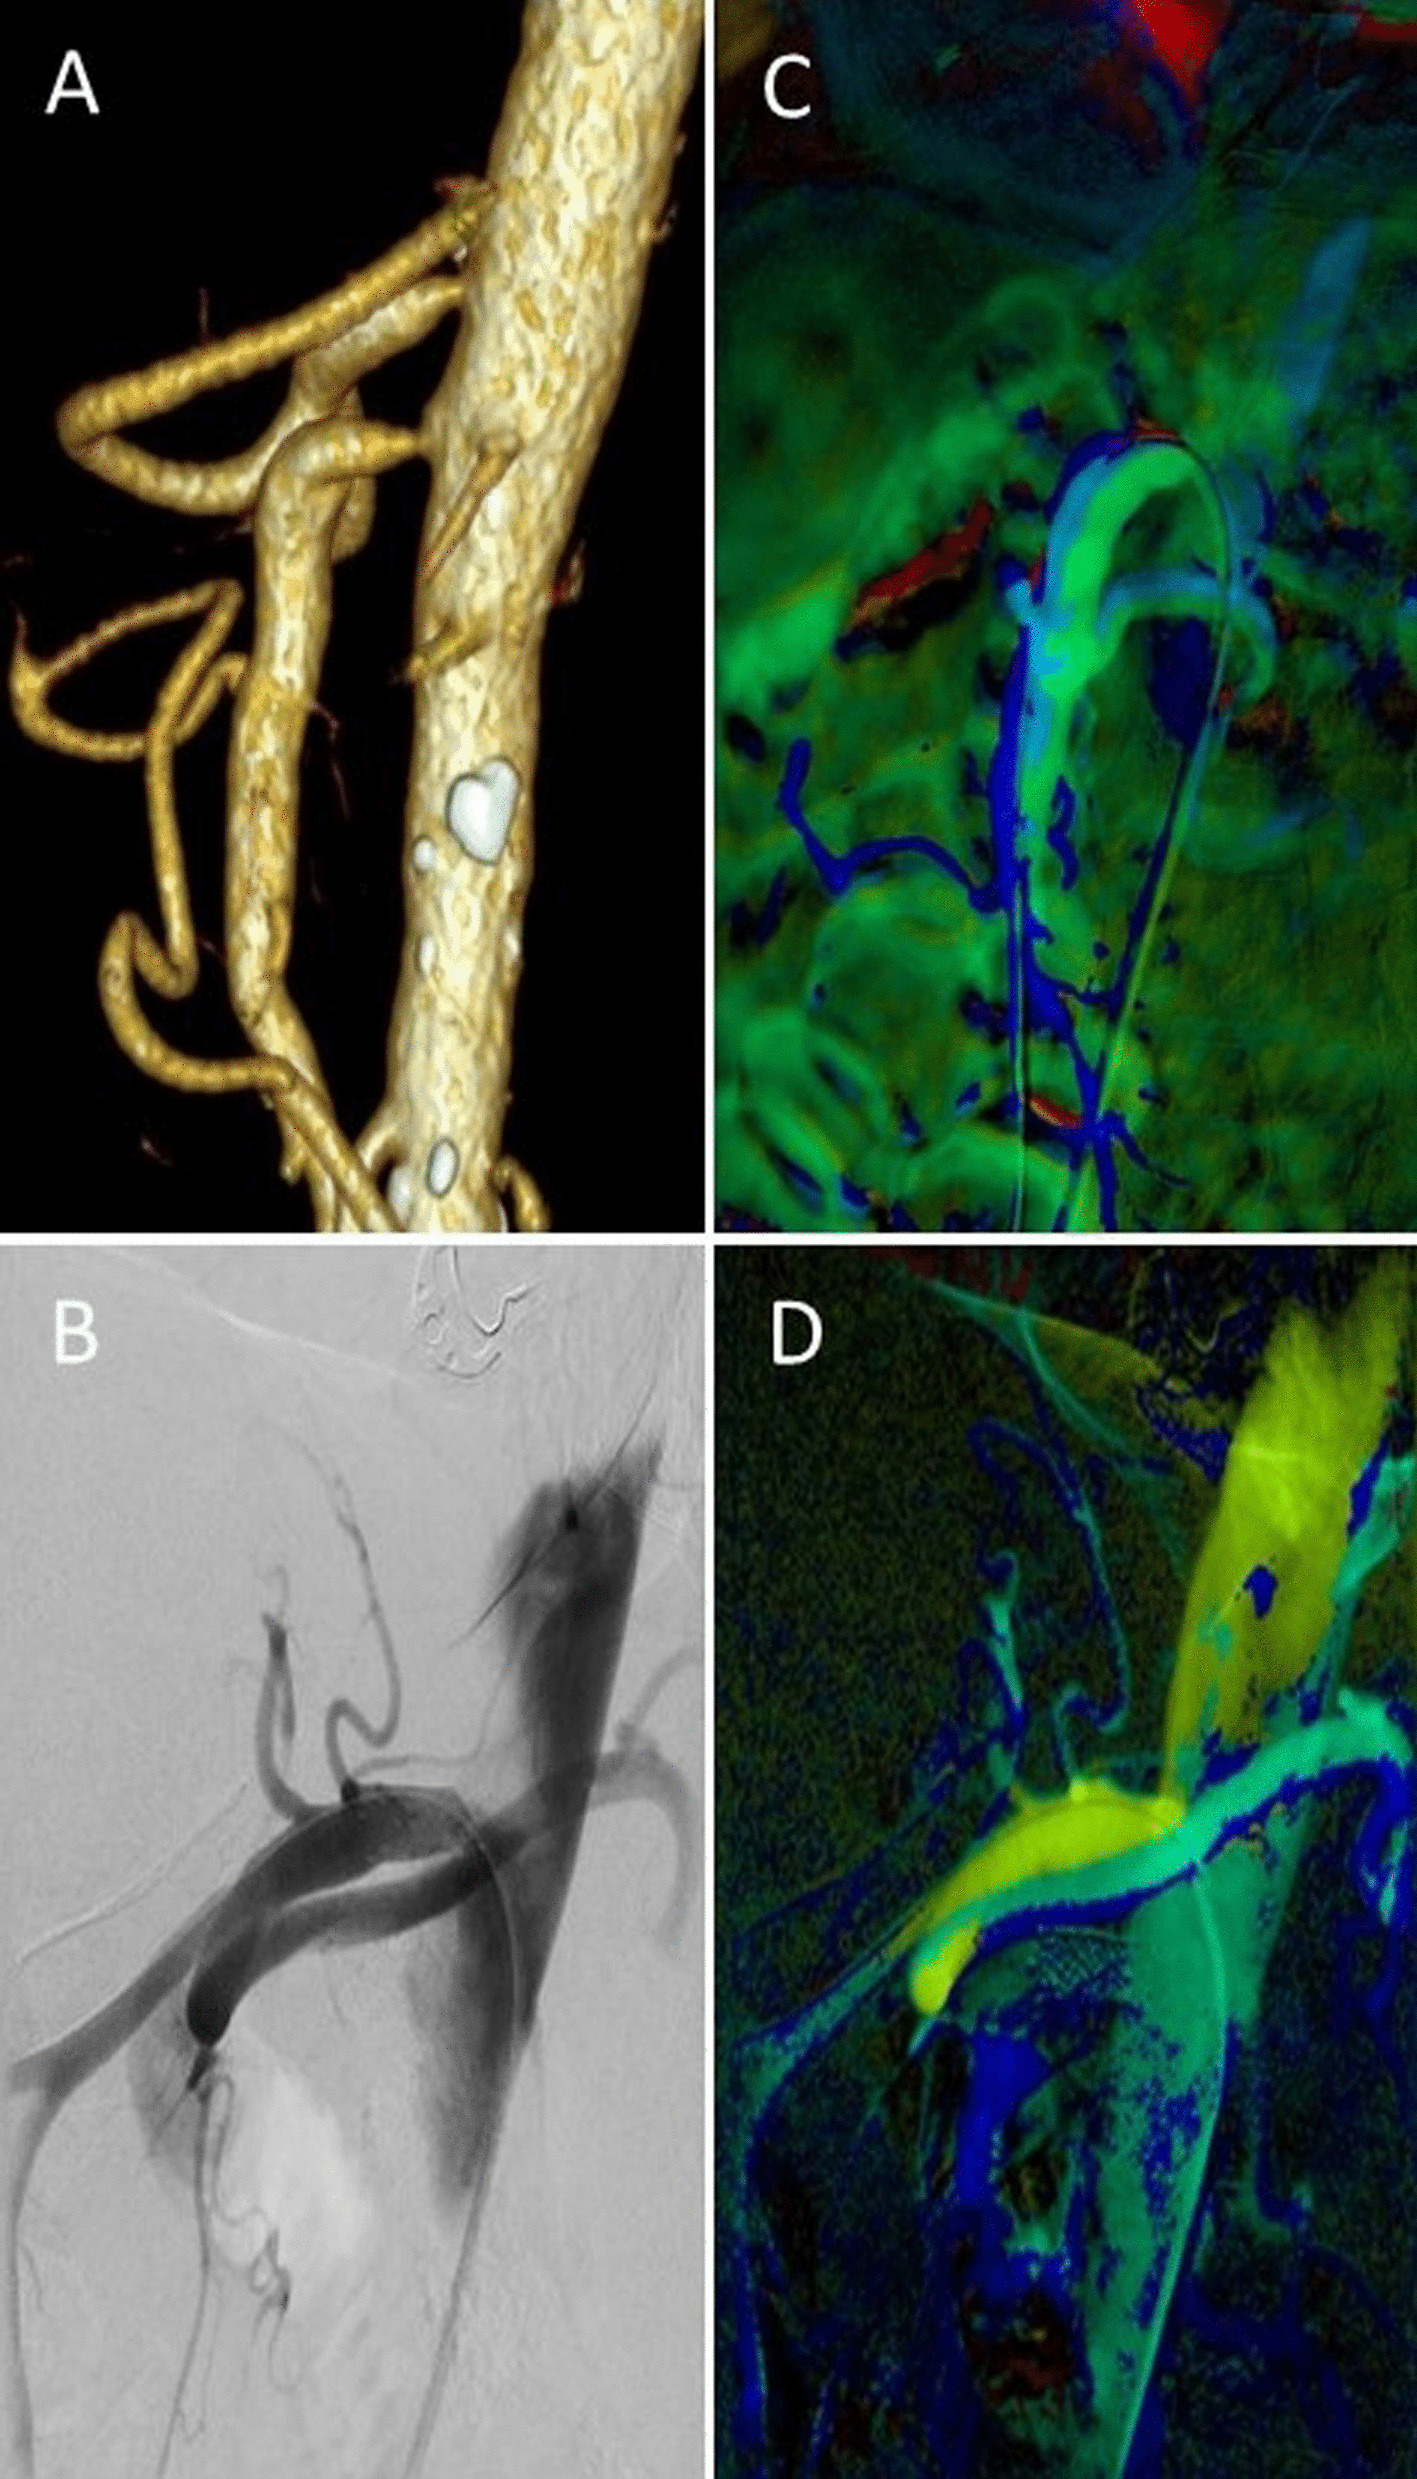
Impaired 2D perfusion assessment in a patient with 2-vessel-disease. Preinterventional Volume Rendering CT Angiography image (A), digital subtraction angiogram and 2D perfusion images showing vessel superimposition due to angulation of the celiac trunk (B, D) and blurring of vessel contours due to breathing artifacts in SMA and celiac trunk (C, D)
